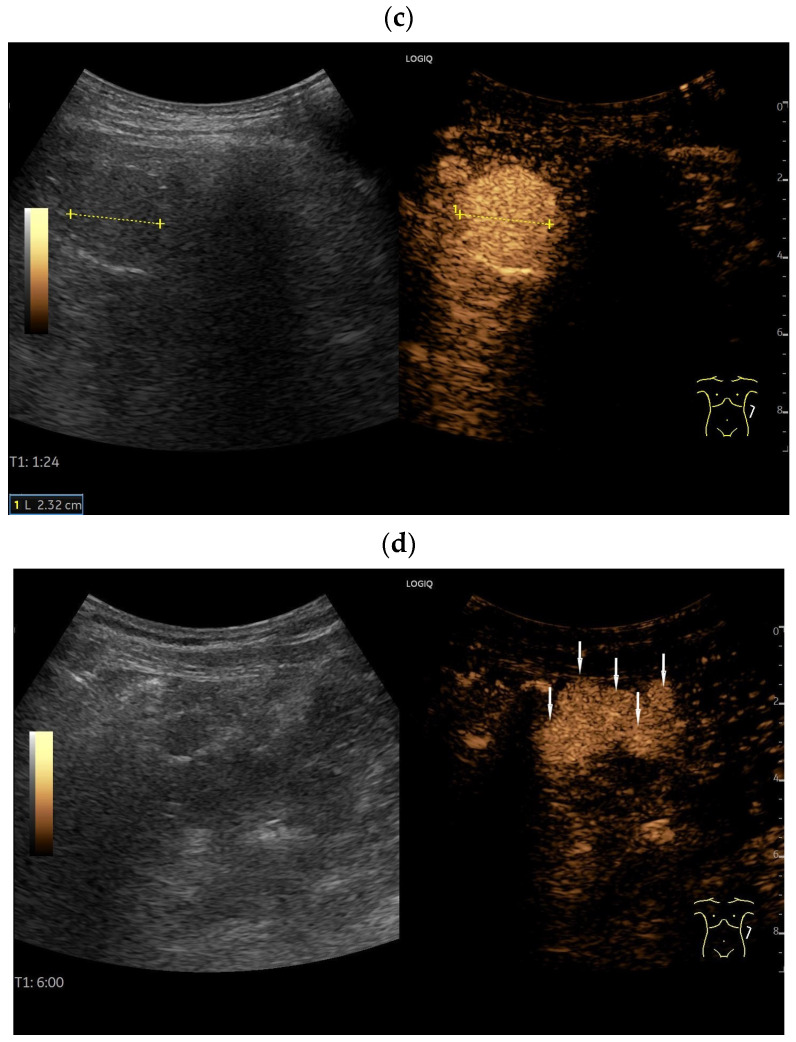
Splenosis. Incidental finding of several round, homogeneous lesions in a patient with a history of post-traumatic splenectomy. The largest lesion, measuring 25 mm (arrow) (a), was adjacent to the left kidney. At least four smaller lesions (arrows), up to 15 mm in diameter, were located interenteric (b) and suggested mesenteric lymphadenopathy. Contrast-enhanced ultrasound (CEUS) using 1.2 mL of SonoVue showed hyperenhancement in all lesions until the end of the examination at 6 min. The larger lesion (between the markers) is visualized after 3 min (c), and the smaller ones (arrows) after 6 min (d). Splenosis was diagnosed in conjunction with the previous splenectomy. No further investigation was necessary.
